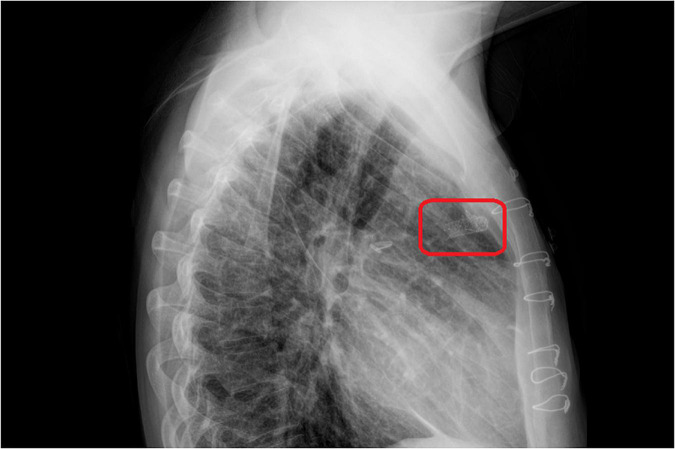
The chest X-ray on the 1 day after stent implantation. The red rectangle showed stent in the central shunt that places in a proper location without mispositioning.
